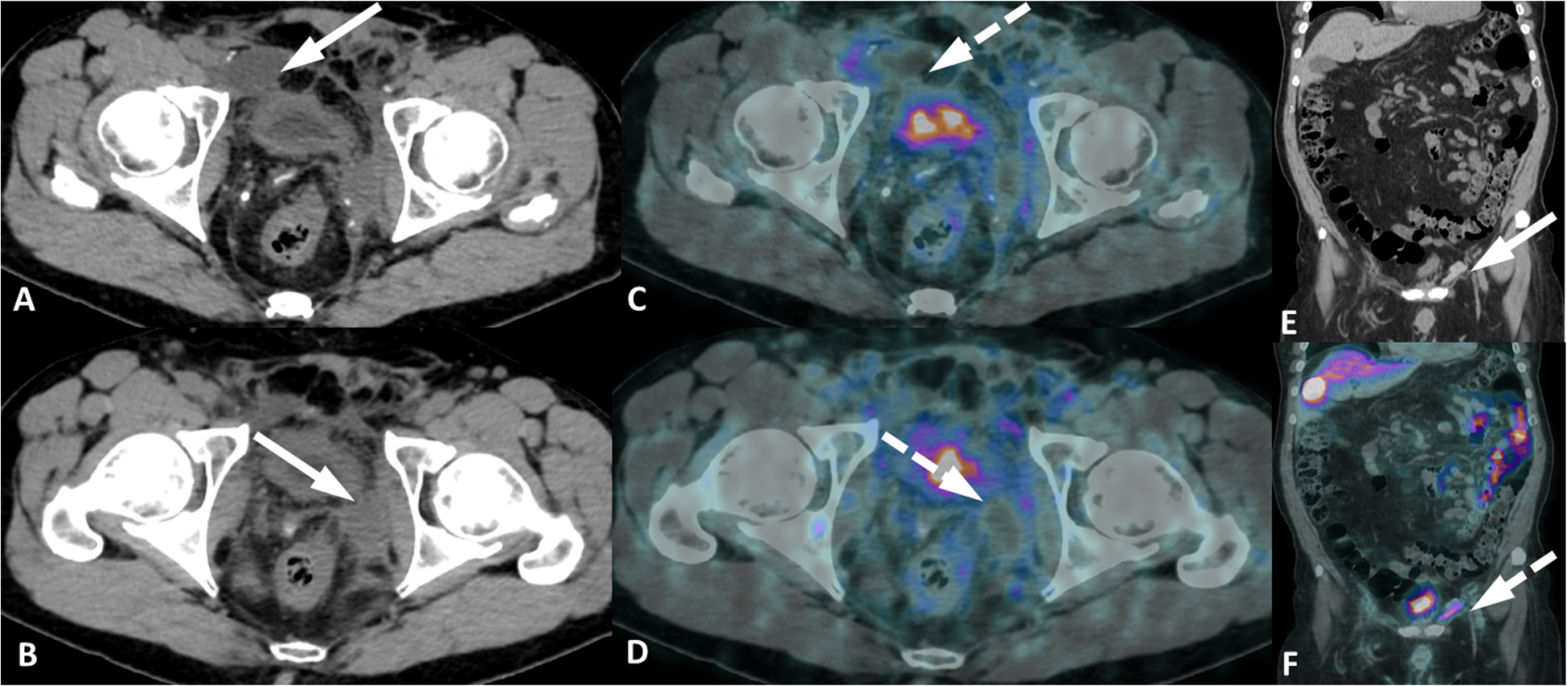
Post-operative inflammatory findings in two patients. Patient 1, prostatic bed inflammation immediate after radical prostatectomy (a-d). Axial CT (a, b) axial PET/CT (c, d) show faint uptake around bilateral lymphoceles (arrows) and inflammatory changes at bilateral pelvic walls (dashed arrows). Patient 2, inflammatory foreign body reaction of mesh material from left inguinal hernia surgery (e, f). Coronal CT (e) and PET/CT (f) images show an elongated hyperattenuating material along the left inguinal canal (arrow) with mild uptake (dashed arrow)
